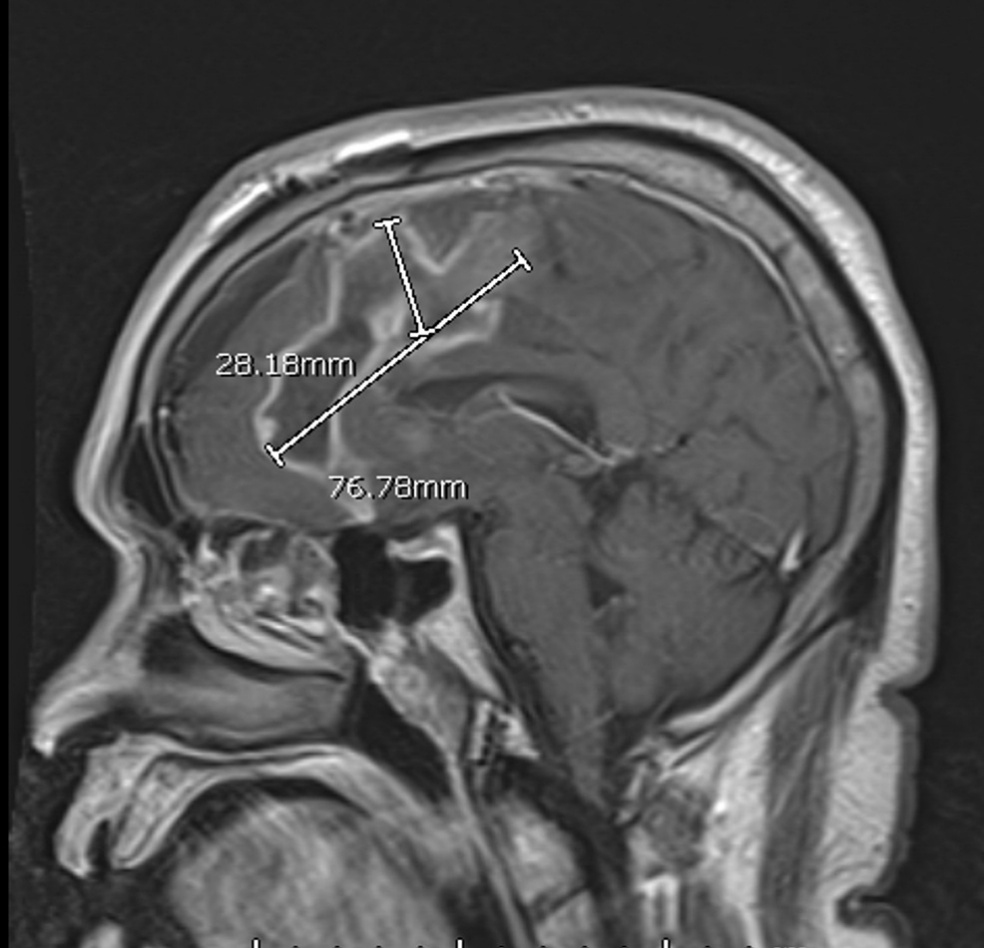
Repeat contrast-enhanced MRI demonstrating a decrease in the size of the abscess cavity. MRI, magnetic resonance imaging
